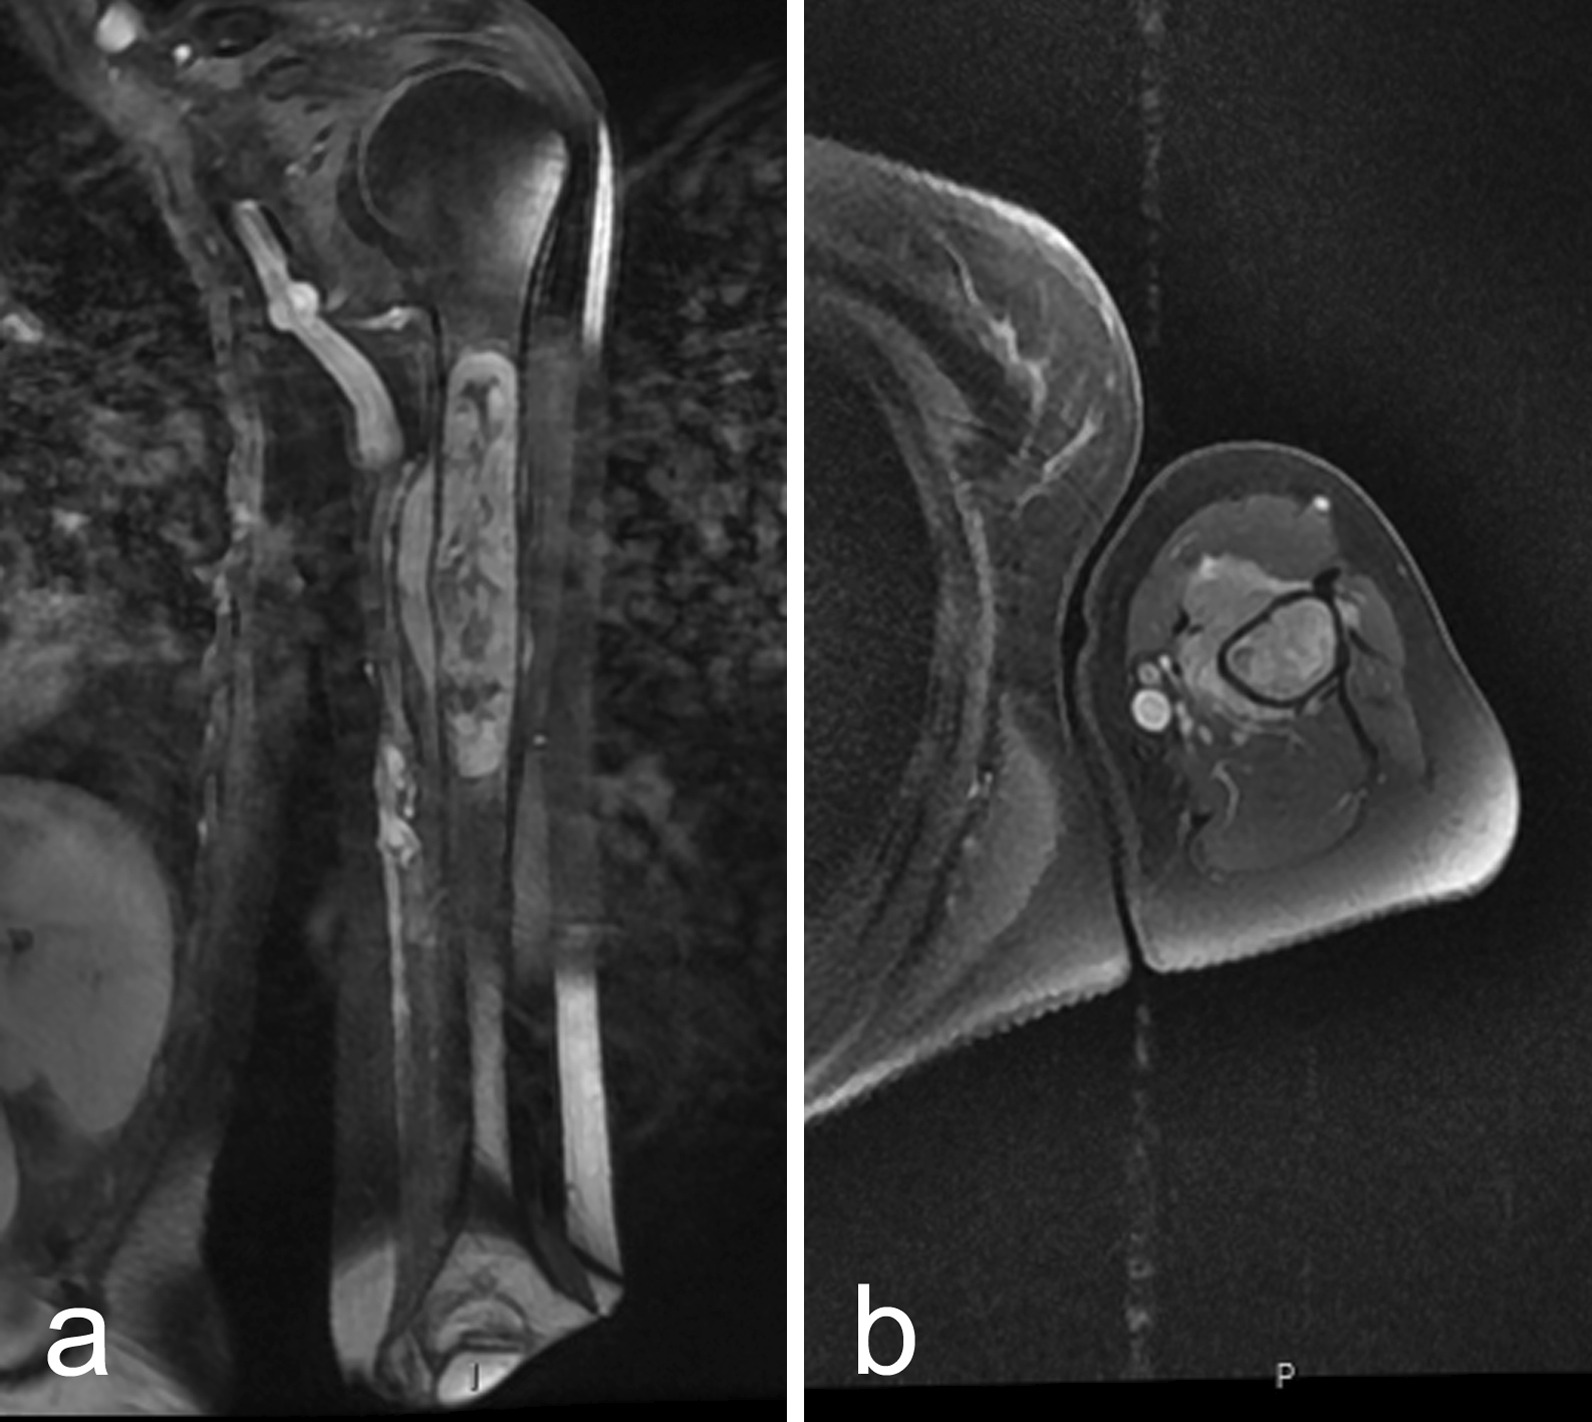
October 2018, magnetic resonance imaging (MRI) scan of the left humerus before treatment. Coronal (a) and axial (b) contrast-enhanced fat-suppressed T1-weighted images. Diaphyseal metastasis with extension to adjacent soft tissue highly enhanced
